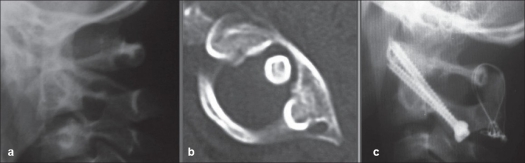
Lateral x-ray of upper cervical spine (a) and CT scan (b) shows type III C1-C2 rotatory subluxation 22 days after injury in a 14 year old girl. The atlanto-dens interval is about 6 mm. she was initially put on skull traction for reduction and then C1-C2 fusion was done with transarticular screws. Followup lateral x-rays (c) shows trasnarticular screw and C1-C2 fusion
